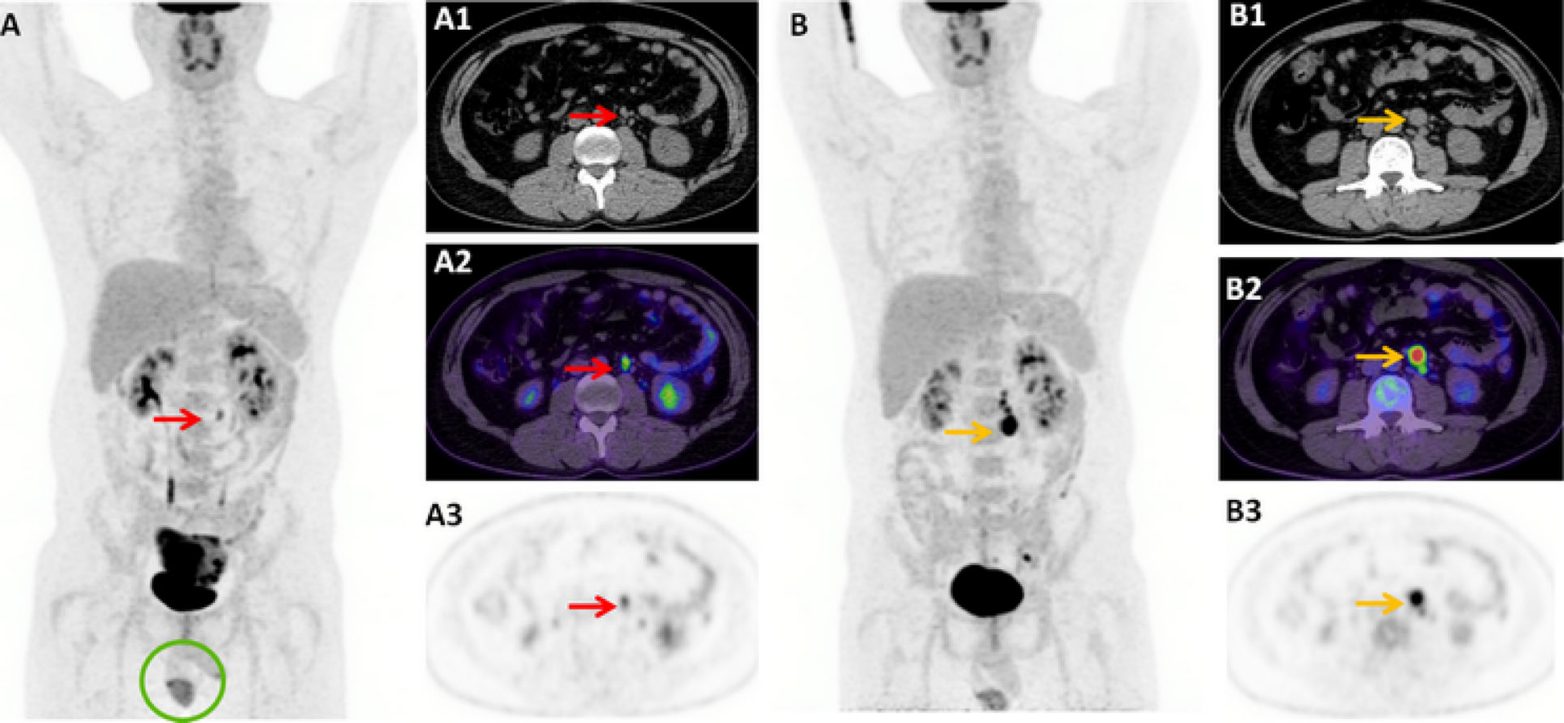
Case Three - Staging FDG-PET/CT demonstrates a bulky and intensely FDG-avid pelvic mass, with absent left scrotal testicular activity [*circle*] (a). A small focus of mild FDG-uptake (SUVmax 4.9) in the upper abdomen corresponds with a 10 mm left para-aortic node on the co-acquired non-contrast enhanced CT [*red arrow*] (a1–3). Re-staging ^18^F-FDG-PET/CT following pelvic resection revealed interval metabolic and structural progression of the left para-aortic node [*orange arrow*] (20 mm; SUVmax 13.4), with a chain of new FDG-avid subcentimetre nodes cranially (B1–3)
